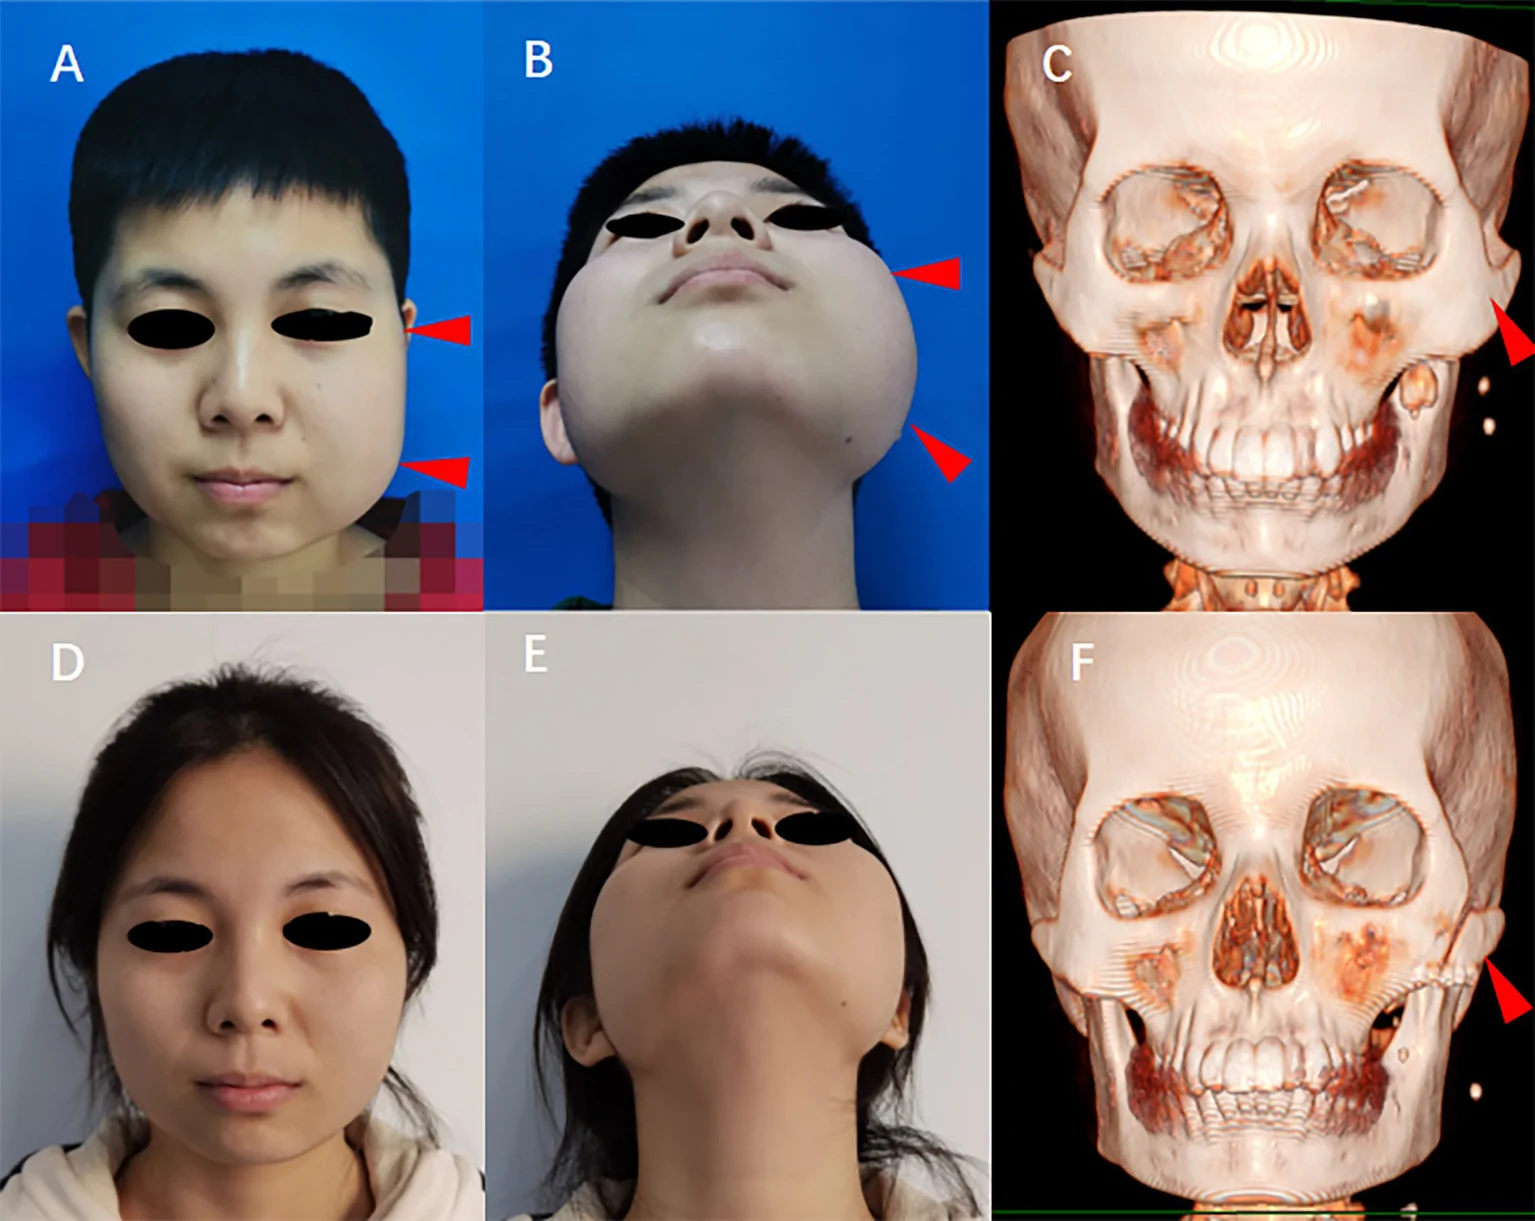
Representative Type III lesion before and after treatment. (A,B) Pre-treatment clinical photographs show facial asymmetry. (C) Pre-treatment three-dimensional CT reconstruction shows zygomatic widening on the affected side. (D,E) Post-treatment clinical photographs show improved facial symmetry. (F) Post-treatment three-dimensional CT reconstruction shows correction of zygomatic asymmetry after contouring osteoplasty.
